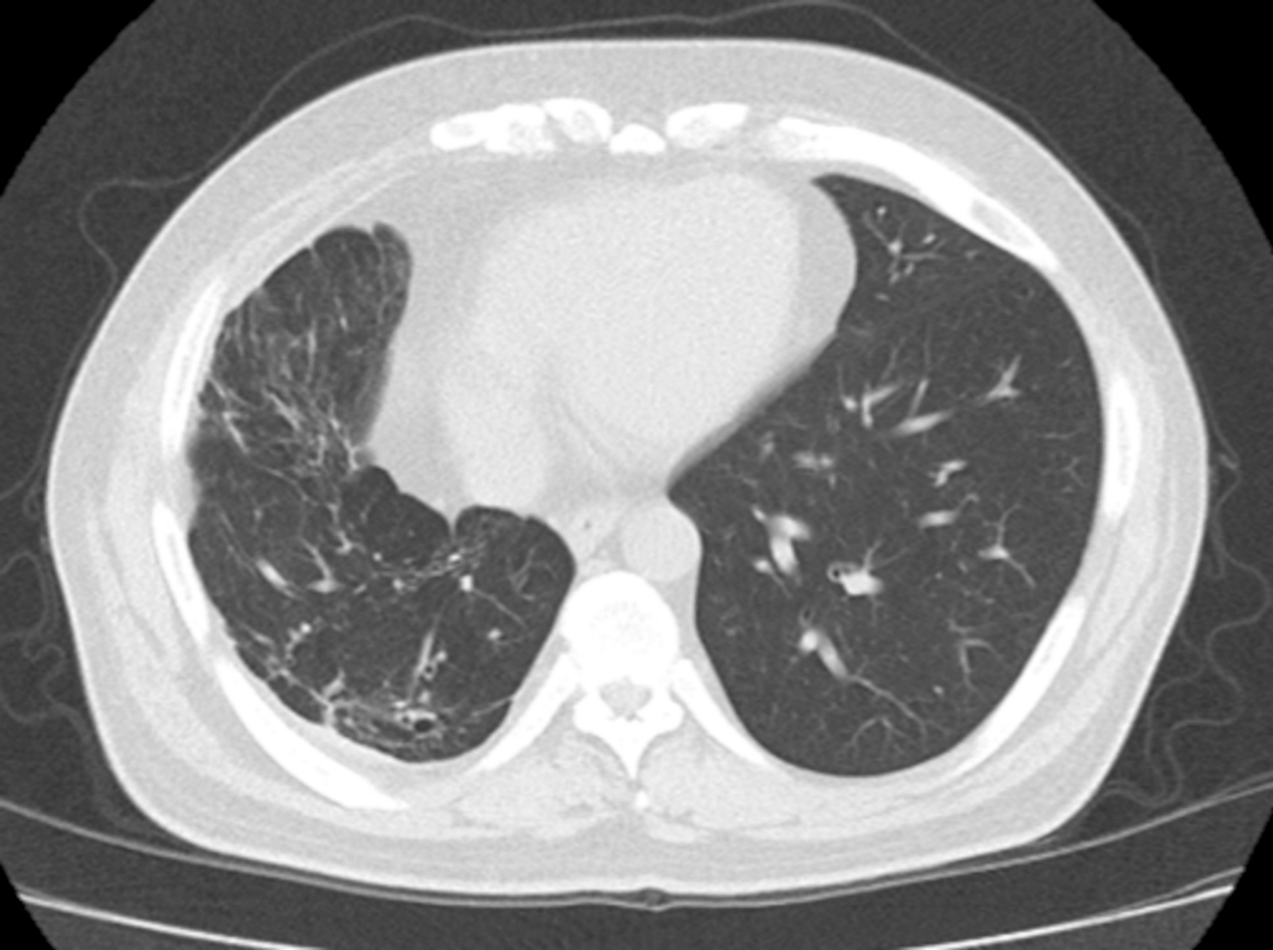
Chest CT findings. Non-contrast chest CT showing no radiographic evidence of pneumonia or pleural effusion.
